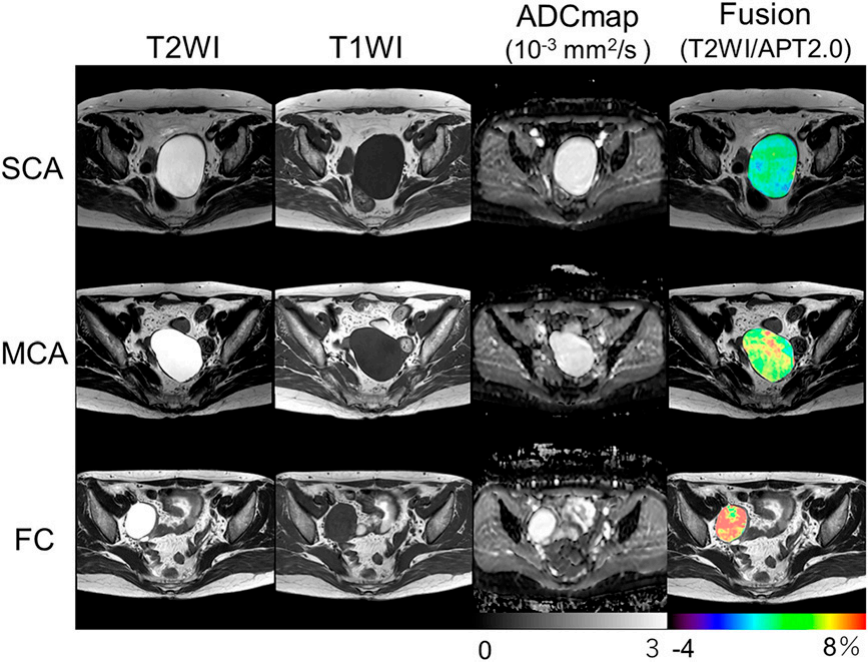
Typical T2-weighted images (T2WI), T1-weighted images (T1WI), apparent diffusion coefficient (ADC) maps, and amide proton transfer maps (APT) (overlaid on T2WI) of serous cystadenoma (SCA) (top), mucinous cystadenoma (MCA) (middle), and functional cyst (FC) (bottom). All 3 cystic lesions showed similar signal intensities in T1WI and T2WI as well as similar ADC values. However, amide proton transfer values were clearly different among trio of cysts: low in serous cystadenoma (2.25%), moderate in mucinous cystadenoma (5.03%), and very high in functional cyst (7.38%). Color bar indicates amide proton transfer signal (%). (Reprinted with permission of (44).)
